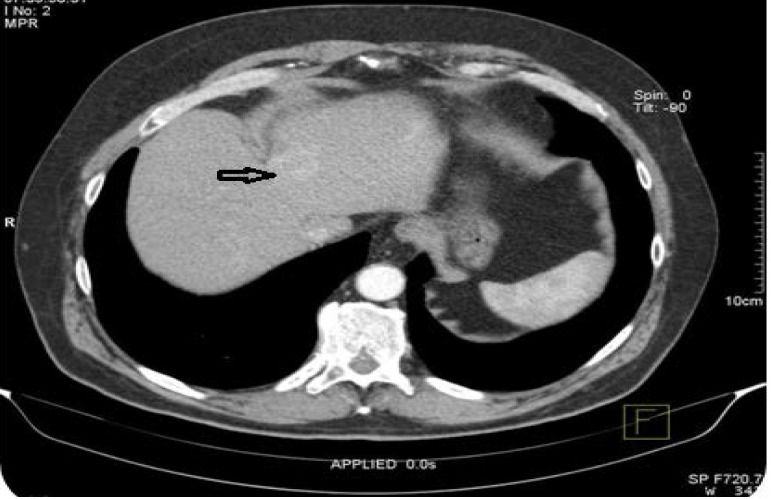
Abdominal computed tomography showing a solid hepatic mass of 3cm x 2,8cm occupying the border between segments II and III (see black arrow)
